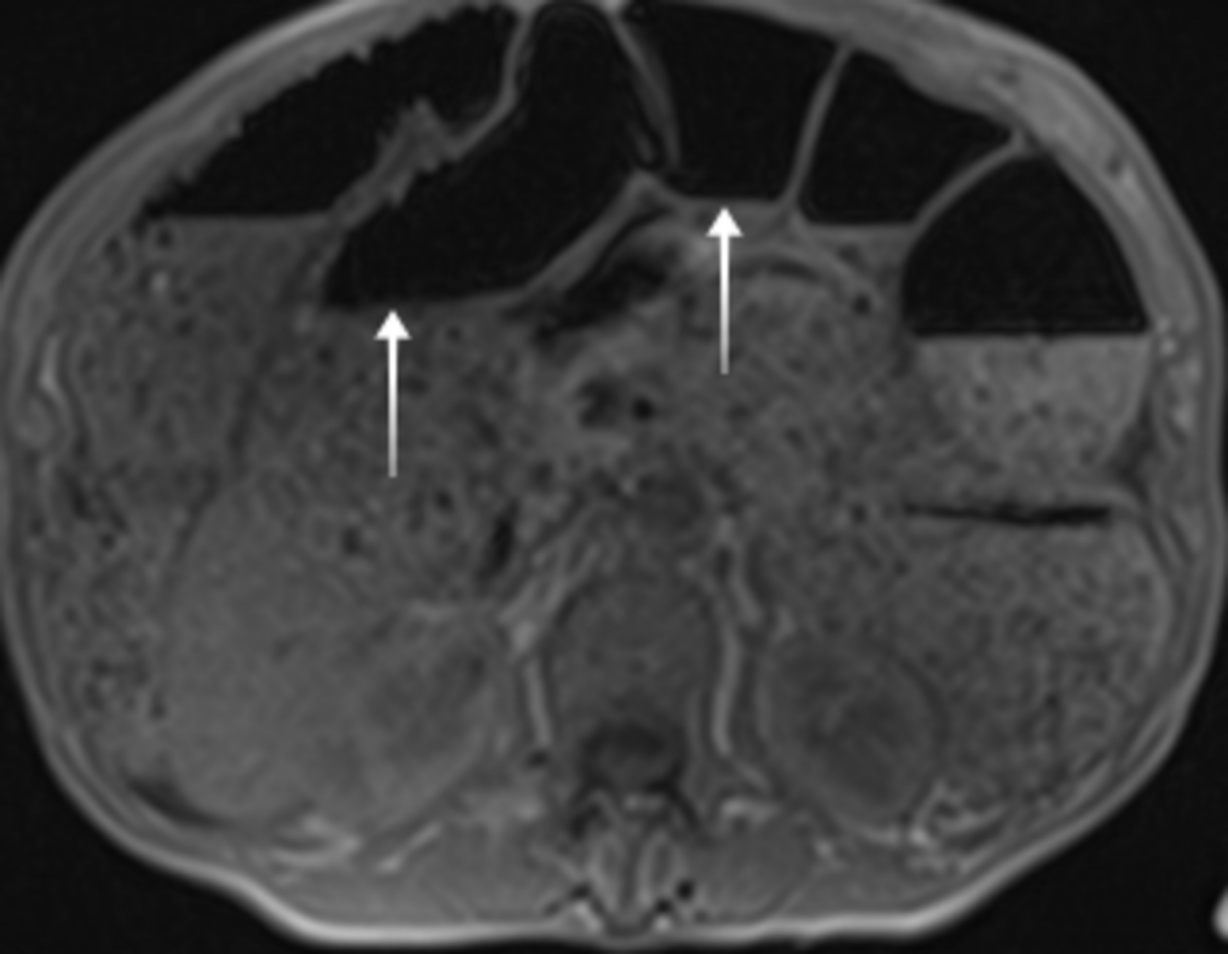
MRI of the abdomen showing distention of large intestine and small bowel with air-fluid levels concerning for distal obstruction. MRI: magnetic resonance imaging
